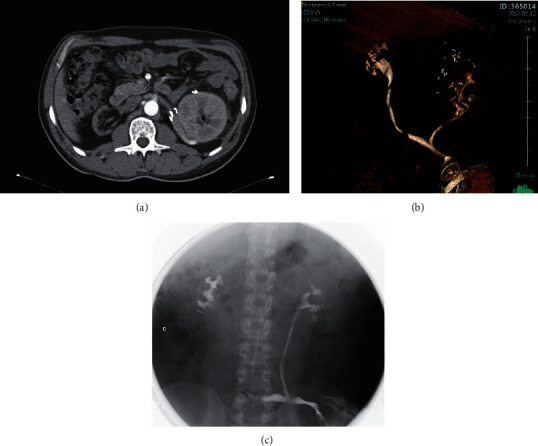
(a). CT scan showing an orthotopic kidney transplantation with the venous reconstruction of the left renal vein. (b) (adapted from Hevia et al.) and (c) are a reconstructed CT image of the urinary system and intravenous urography, respectively, representing a ureteroureterostomy in orthotopic renal transplantation in a patient with a history of myelomeningocele and cystectomy with cutaneous ureterostomy.
